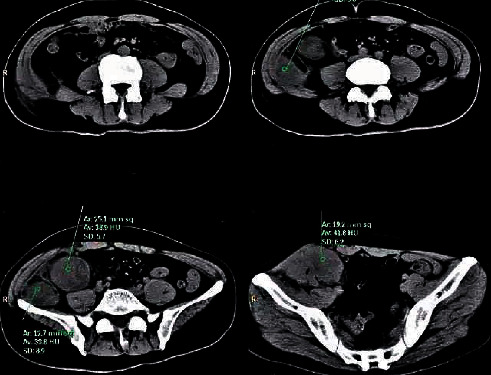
CT image of a 50-year-old male patient complaining about repeated right and lower abdominal pain with abdominal mass for over two months.
